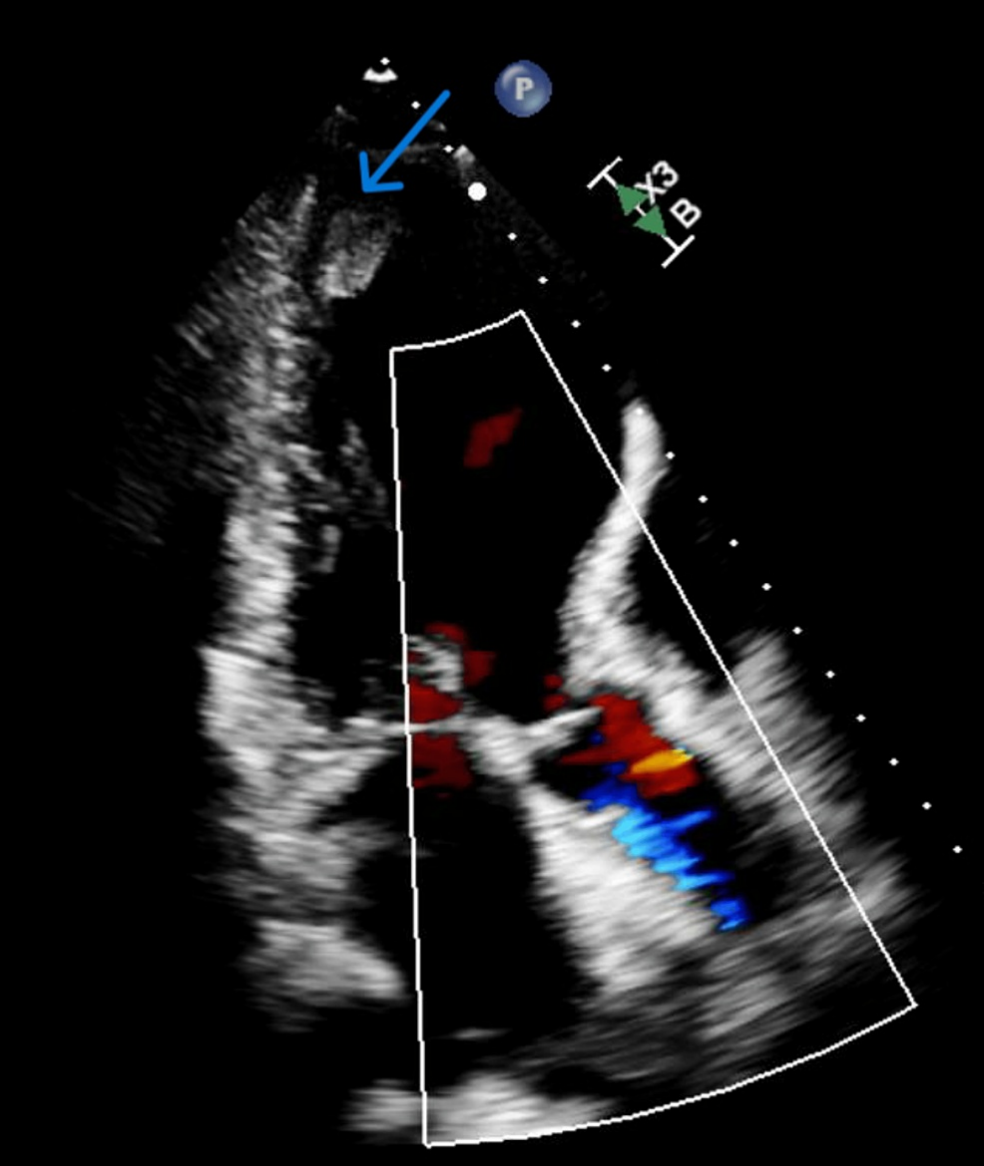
Transthoracic echocardiogram The blue arrow points to a large thrombus at the apex of the left ventricle.
